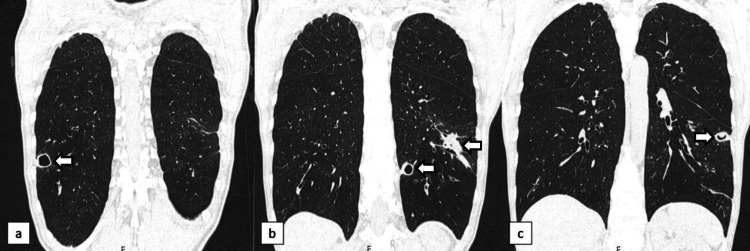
CT scan two weeks after completing antibiotic therapy for pneumocystis pneumonia Images a-c correspond to coronal sections revealing some cavitated nodules with variable dimensions, with typical features of GPA GPA, granulomatosis with polyangiitis
